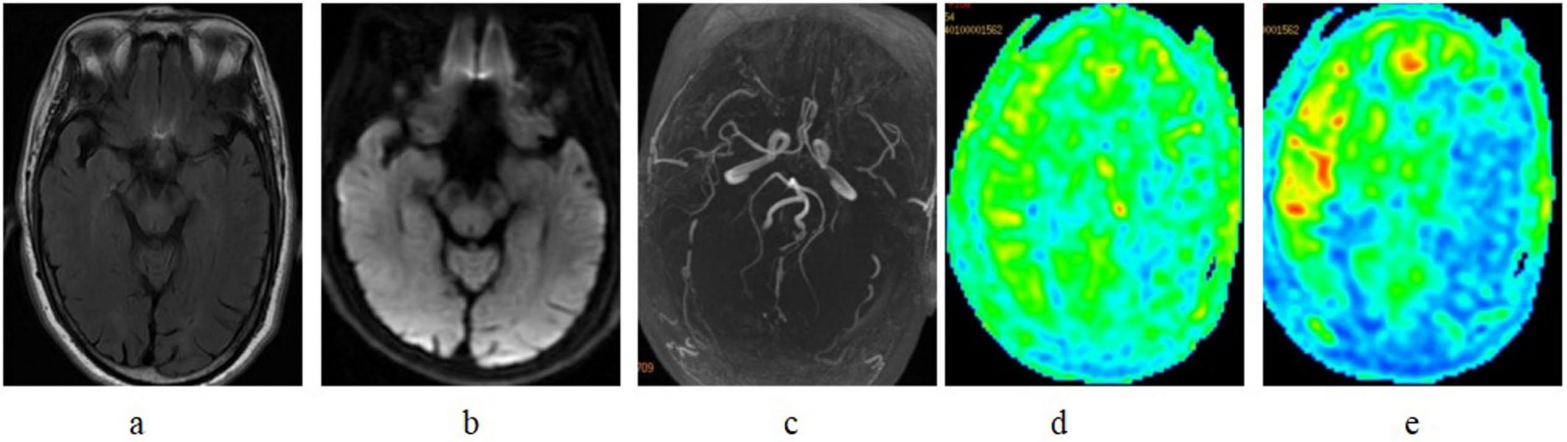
FLAIR vascular hyperintensity in a patient with left middle cerebral artery occlusion. (a) Serpentine hyperintense signal of the middle cerebral artery branches in the left sylvian fissure in T2 FLAIR. (b) DWI demonstrate negative finding. (c) MRA showed occlusion of the left MCA M1. (d) On ASL with a PLD of 1525 ms markedly decreased ASL signals are noted in the extended area of the left hemisphere. ASL with a PLD of 2525 ms, (e) the decreased area is somewhat improved, but there is still a slight laterality compared with the right side.
